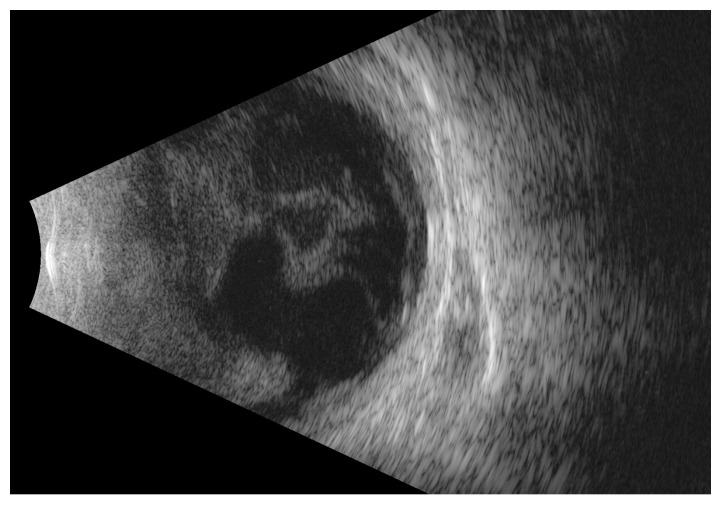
B-scan ultrasound of the left eye shows progression with a rapid increase in hyperechoic material in the vitreous chamber after 24 h.
